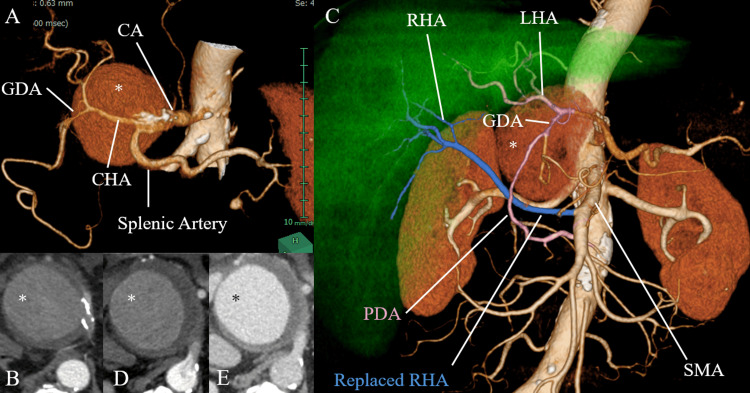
Preoperative CT findings Asterisks indicate celiac artery aneurysm. (A, B) Celiac artery aneurysm arising from a calcified celiac artery; (C) Three-dimensional reconstruction image of hepatic circulation; (D) Retrograde blood flow in the celiac artery during the arterial phase; (E) Contrast enhancement of the celiac artery in the venous phase. CA: celiac artery; CHA: common hepatic artery; GDA: gastroduodenal artery; RHA: right hepatic artery; LHA: left hepatic artery; SMA: superior mesenteric artery; PDA: pancreaticoduodenal arcade.
